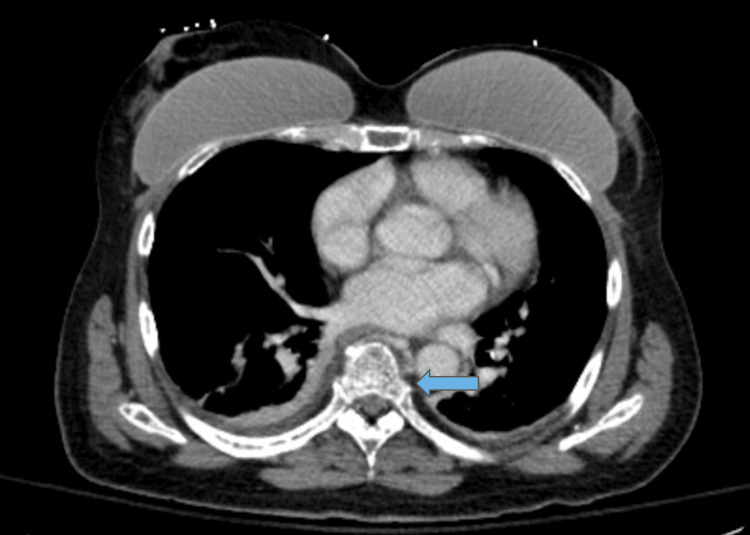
CT chest (axial view) demonstrating generalized osteopenia and lytic lesions in the thoracic spine likely due to multiple myeloma, less likely metastatic disease (blue arrow).
